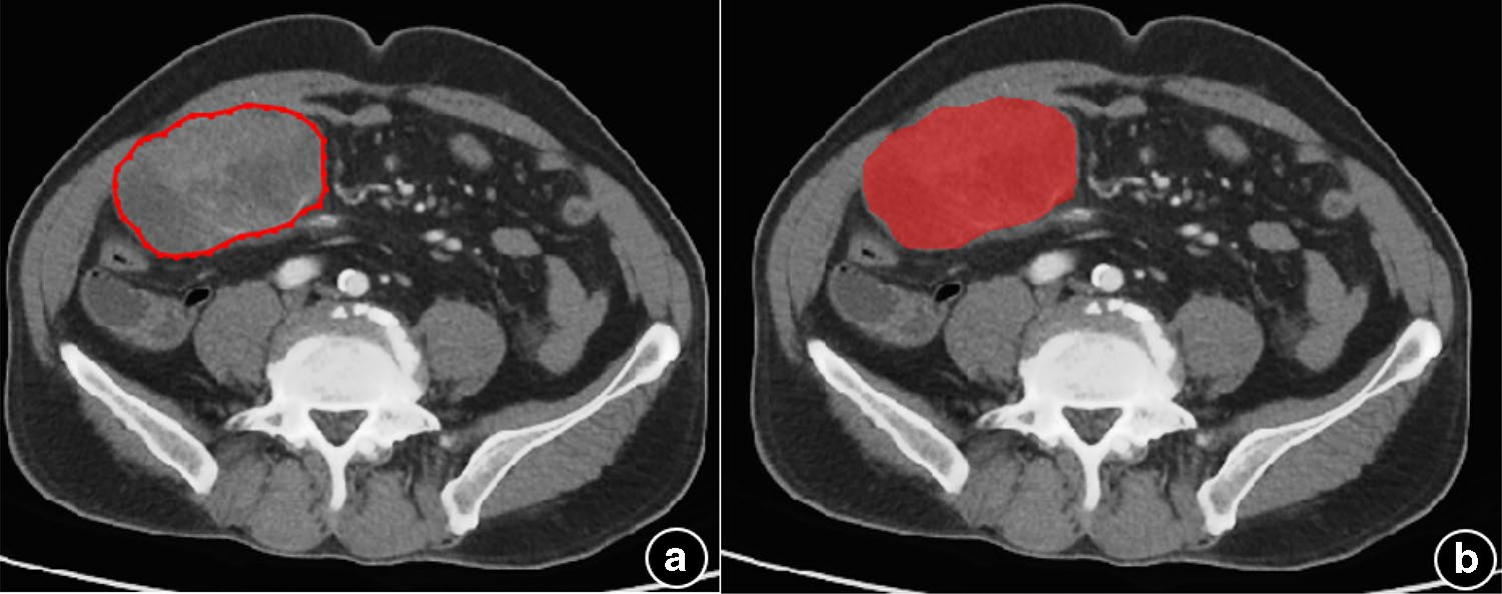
The image-processing software ITK⁃SNAP was used to manually delineate tumor ROIs along the lesion fringes on all the layers containing the tumors through the enhanced-CT venous phase images, and then the images were merged into 3D ROI images (red).
